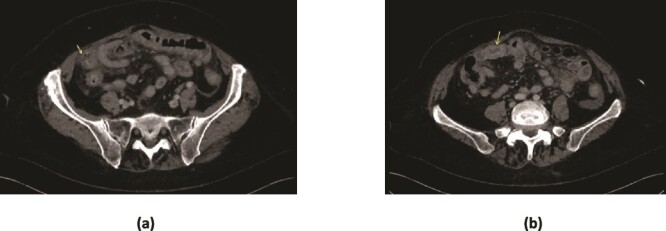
CT scan demonstrating (a) marked mural thickening of the small bowel in the right lower quadrant with areas of mural discontinuity (arrow) and peritoneal free fluid suggestive of perforation (b) ovoid soft tissue density in a small bowel loop, with collapsed small bowel loops distally.
